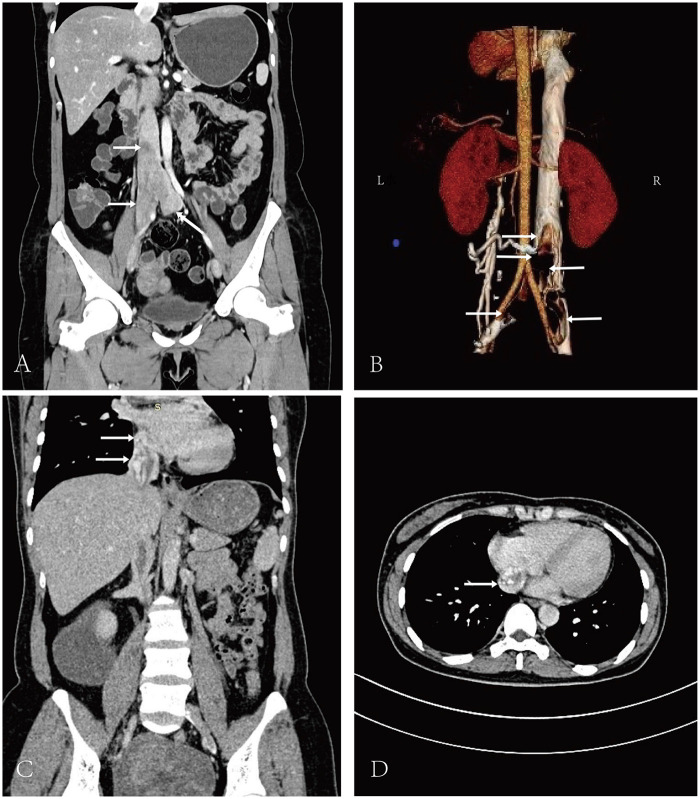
Computed tomography venography image of intravenous leiomyomatosis with inferior vena cava, iliac veins, or intracardiac extension. (A) Tumors in the inferior vena cava and bilateral common iliac veins (arrows) in Patient 1. (B) Tumors in the inferior vena cava and bilateral common iliac veins presenting filling defects in the corresponding vascular sites (arrows) in Patient 1. (C) The tumor in the inferior vena cava extends to the right atrium (arrows) in Patient 5. (D) Tumor in the right atrium (arrows) in Patient 5.
